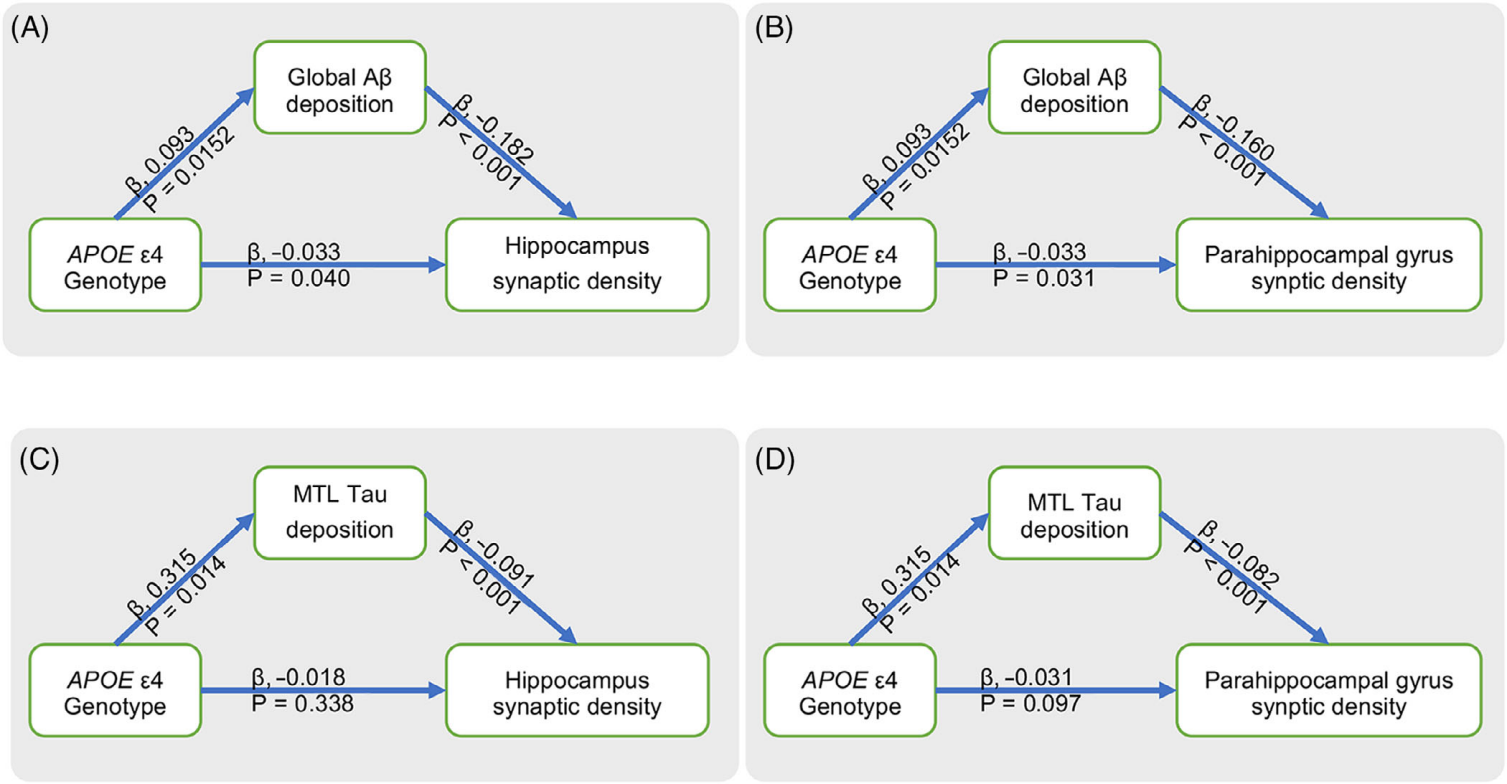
Mediation effects of Aβ/tau pathology on relationship between *APOE* ε4 genotype and synaptic density. (A, B) Mediating effects of global Aβ PET SUVr on association between *APOE* ε4 genotype and synaptic density in hippocampus (indirect effects = −0.017, SE = 0.008, 95% CI = −0.036 to −0.003; direct effects = −0.033, SE = 0.016, 95% CI = −0.066 to −0.002) and parahippocampal gyrus (indirect effects = −0.015, SE = 0.007; 95% CI = −0.031 to −0.003; direct effects = −0.033, SE = 0.015, 95% CI = −0.063 to −0.003). (C, D) Mediating effects of medial temporal cortex tau PET SUVr on relationship between *APOE* ε4 genotype and synaptic density in hippocampus (indirect effects = −0.029, SE = 0.011, 95% CI = −0.052 to −0.008; direct effects = −0.018, SE = 0.019, 95% CI = −0.056 to 0.020) and parahippocampal gyrus (indirect effects = −0.026, SE = 0.010, 95% CI = −0.046 to −0.007; direct effects = −0.031, SE = 0.018, 95% CI = −0.067 to 0.006).
